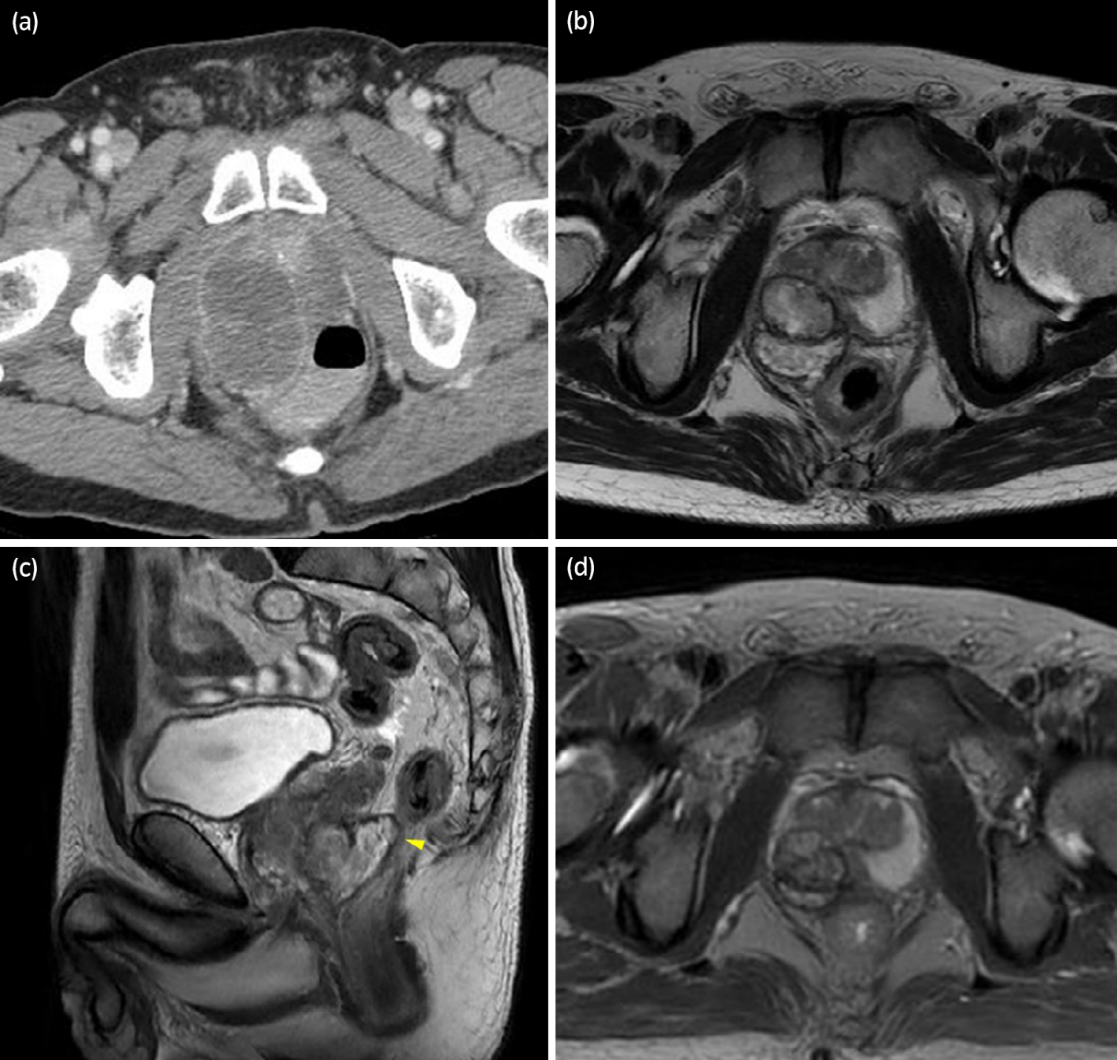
(a) Contrast‐enhanced CT shows a 62 × 40 mm^2^ mass in the right prostatic lobe. (b, c) MRI after two courses of CTh shows a 48% reduction in tumor size and suggests rectal invasion (yellow arrowhead). (d) MRI after four courses of CTh shows a 75% reduction in tumor size from the initial examination.
